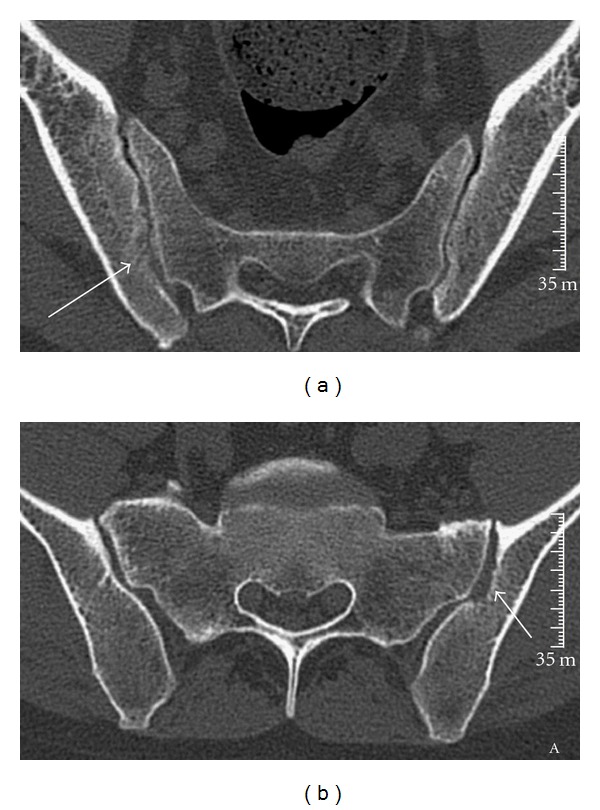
(a) CT scan demonstrates erosions on the right SIJ (*arrow*). (b) CT scan demonstrates erosions on the left SIJ (*arrow*).
